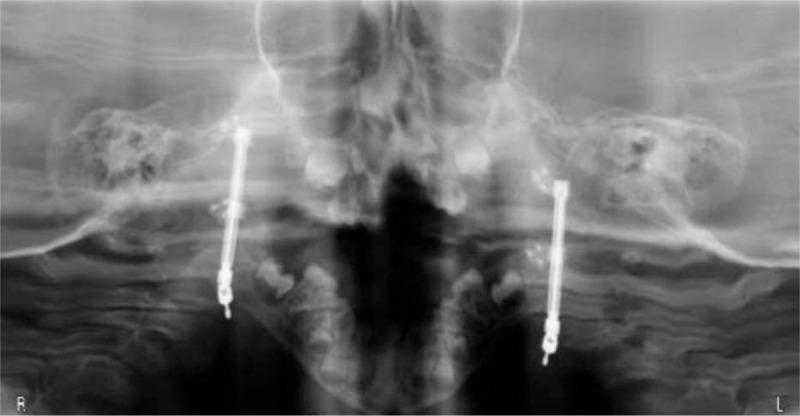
CT scan after 17 days of distraction. The superior portion of the right-sided distractor is in the glenoid fossa.
